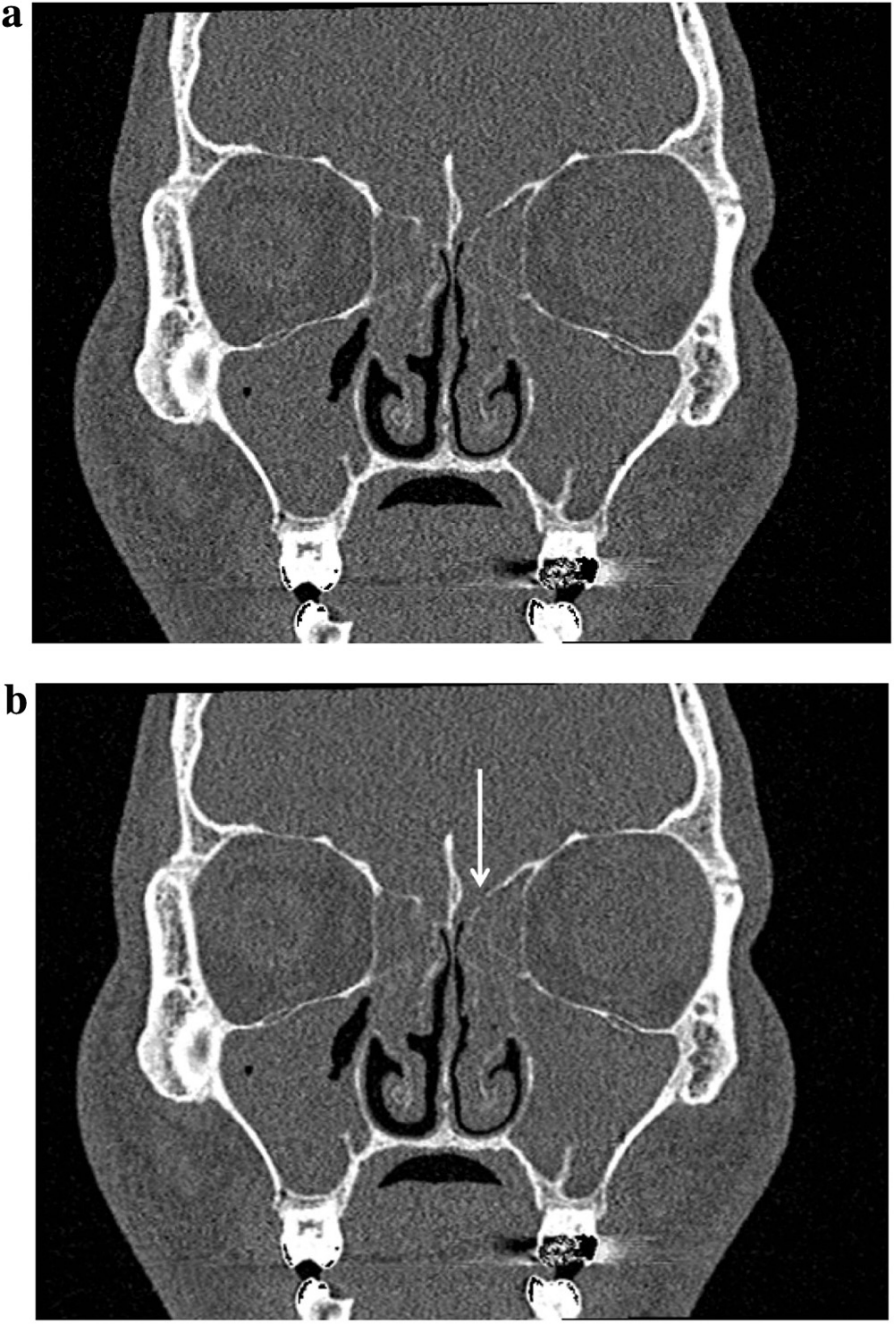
(a) CT Sinuses showing area of bony erosion in the left aspect of the cribriform plate (unmarked). (b) CT Sinuses showing area of bony erosion in the left aspect of the cribriform plate (marked).
